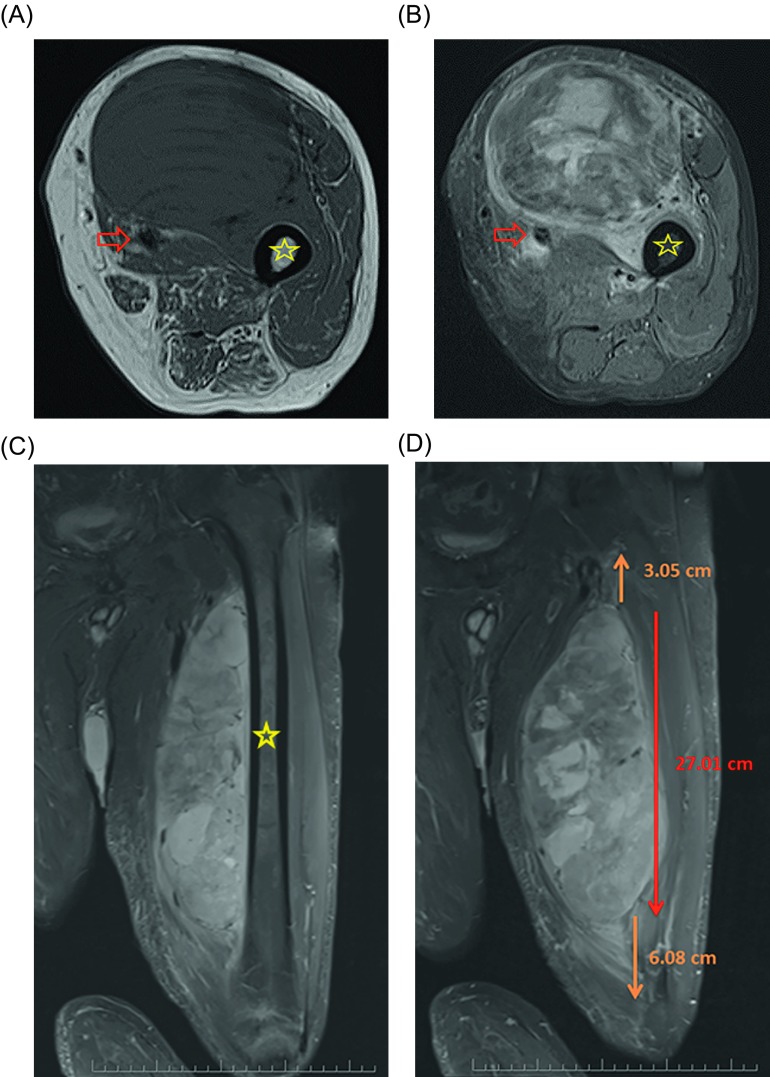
Selected T1 (A) and fat-saturated T2 (B) axial as well as fat-saturated coronal T2 (C) MRI images of a 60-year-old patient with a large, deep mass located in the anterior thigh. On the pretreatment imaging the mass was intimately associated with the femoral neurovascular bundle (arrow) as well as the periosteum of the femur (star). A biopsy was performed and showed high-grade pleomorphic rhabdomyosarcoma. The mass measured approximately 27 cm cranial/caudal however was associated with peritumoral edema which spanned nearly the entire length of the femur on coronal fat-saturated T2 (D) MRI images.
